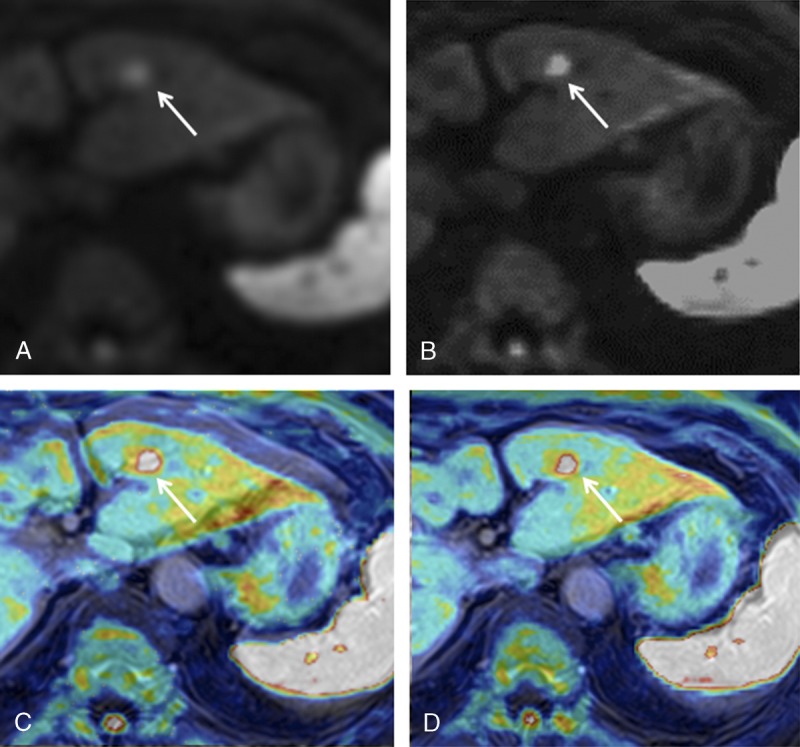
HCC in an 82-year-old woman. A, cc-DWI; B, hc-DWI; C, cc-DWI (color image) fused onto the T1WI obtained during PVP (grayscale image); D, hc-DWI (color image) fused onto the T1WI acquired during PVP (grayscale image). The HCC (arrow) is more clearly depicted on the hc- than the cc-DWI. Note displacement of the liver surface on the cc-DWI and the T1WI scan. The mis-registration on the cc-is corrected on the hc-DWI. Figure 6 can be viewed online in color at www.jcat.org.
